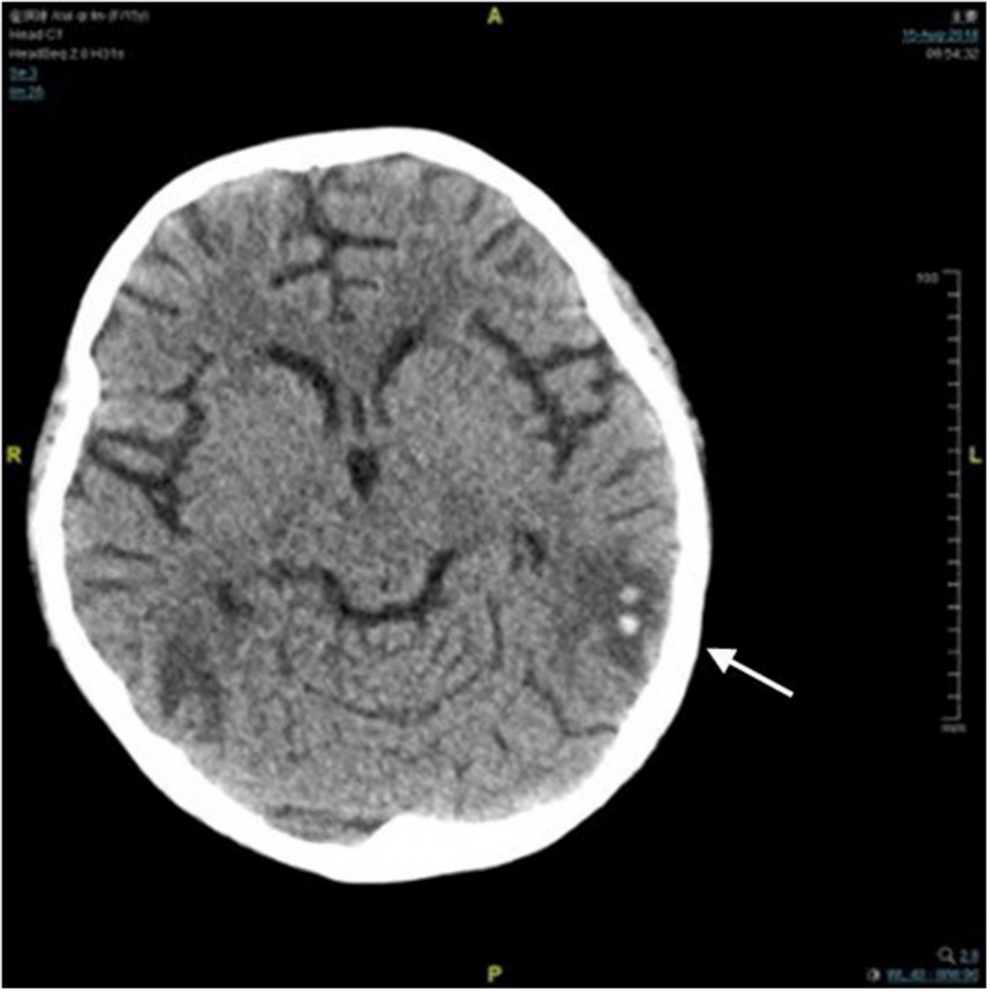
Computed tomography image of brain. There was a small amount of hemorrhage in the left temporal lobe (arrow).
